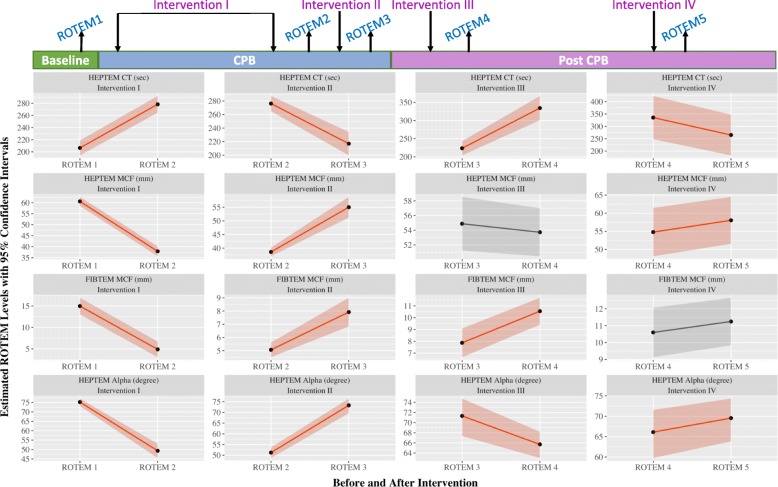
Estimated ROTEM values before and after interventions. Intervention means each waves of administration of blood products. CPB pre-platelets were prime CPB PRBC, prime CPB platelets and prime CPB FFP. CPB post-platelets included run CPB PRBC, run CPB platelets and run CPB FFP. Post bypass HFC was HFC only. Post-bypass platelets included HFC, platelets, CS, FFP, PRBC, Cryo and PB. Estimates before and after each intervention were predicted marginal means. Significant changes in ROTEM levels were marked in red. CPB, cardiopulmonary bypass; CS, cell saver; CT, clotting time; FFP, fresh frozen plasma; HFC, human fibrinogen concentrate; MCF, maximum clot firmness; PB, phlebotomized blood; PRBC, packed red blood cells
